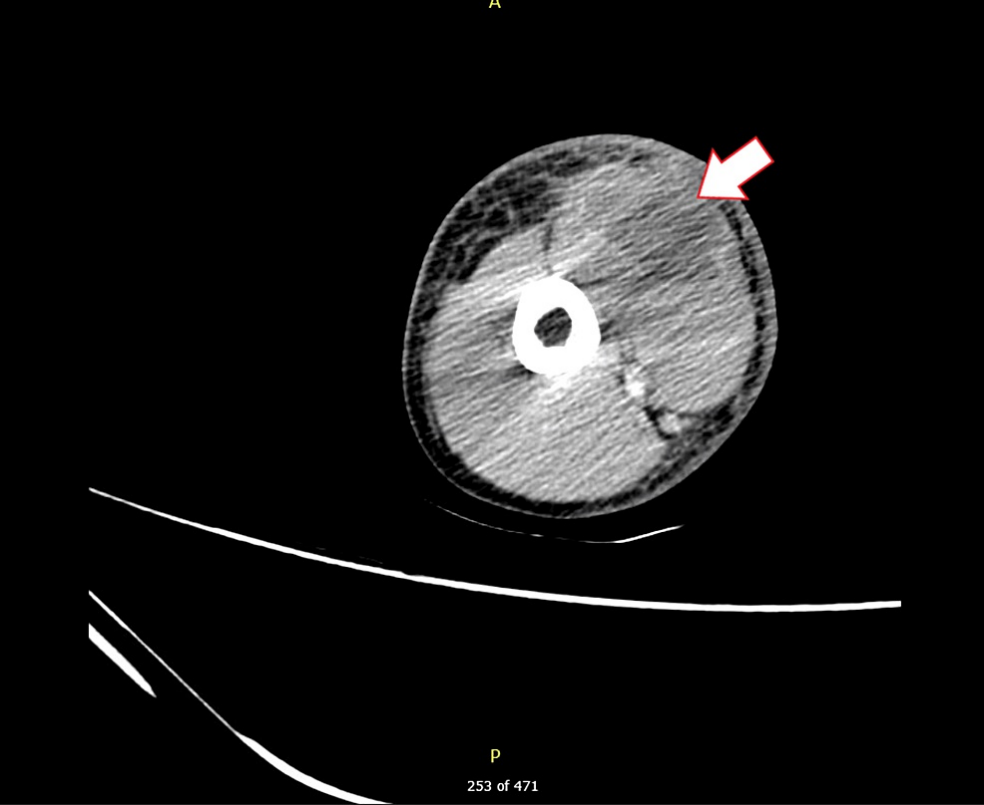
Computed tomography with intravenous contrast of the left upper extremity displaying a 2.3 × 3.4 × 4.8 cm collection contained within the biceps brachii
